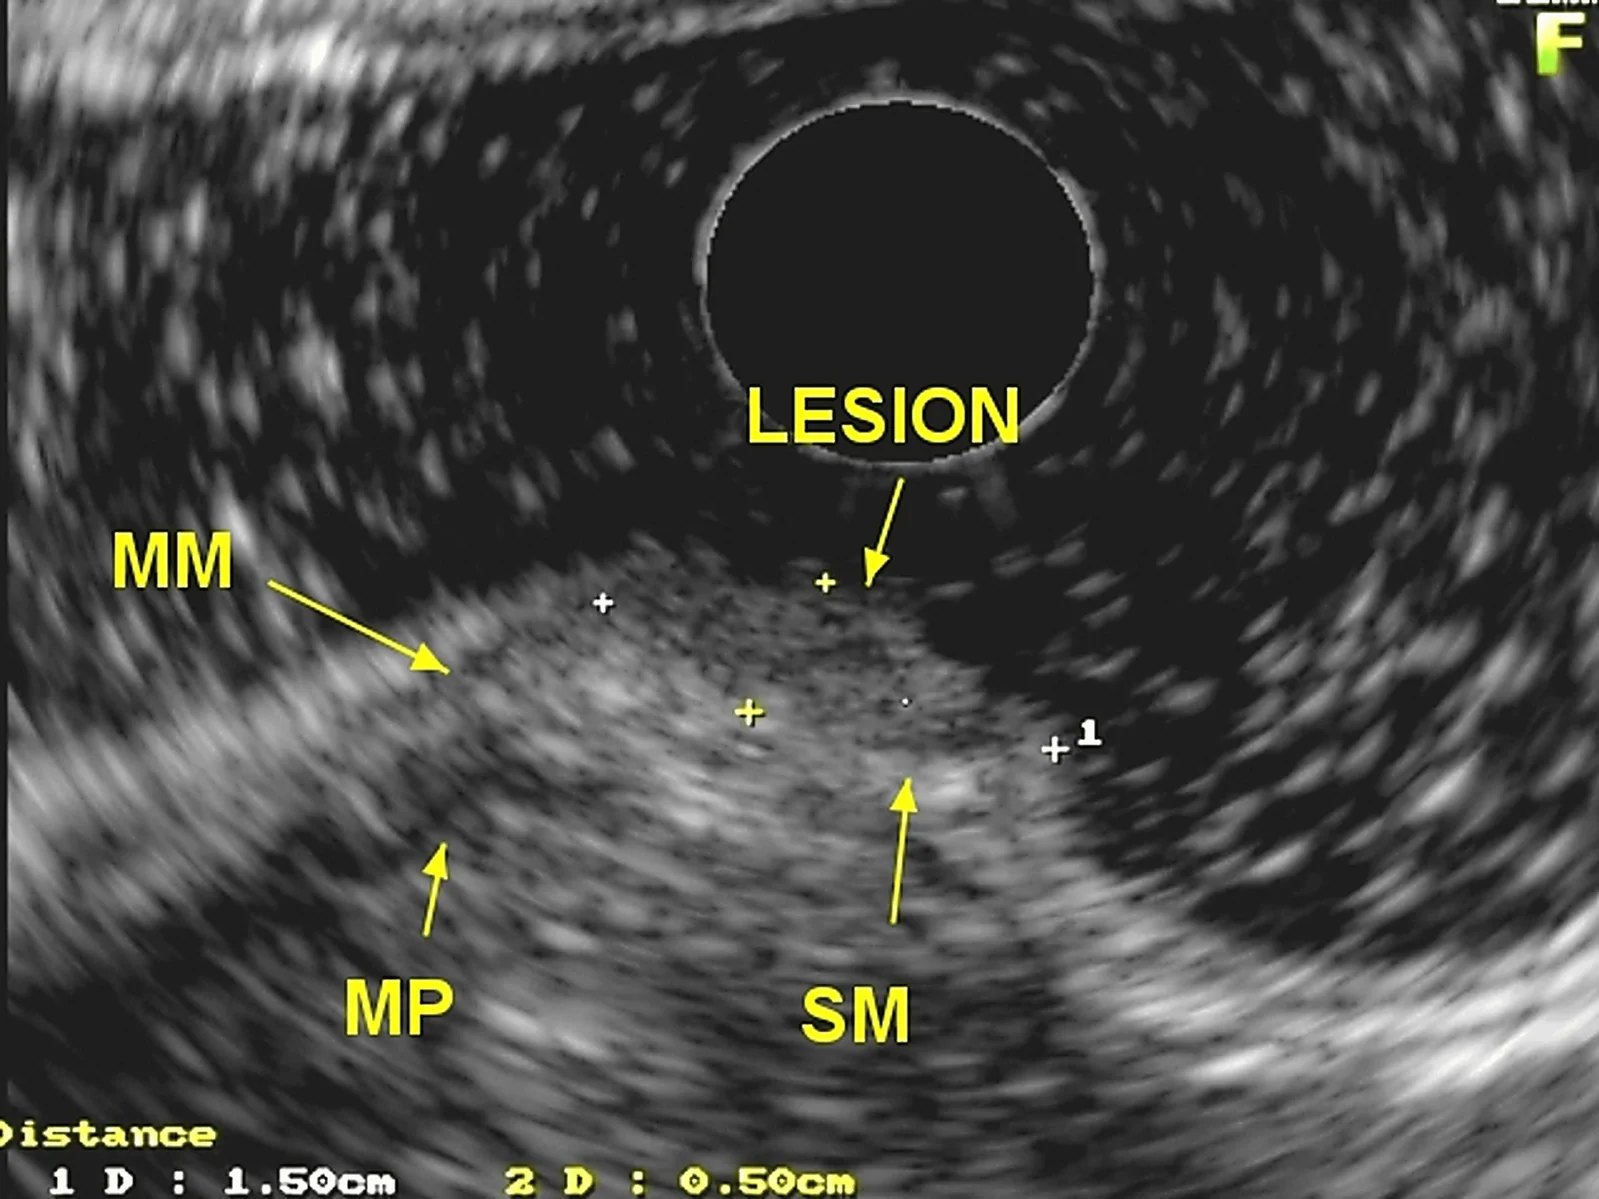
Radial EUS showing a hypoechoic gastric lesion involving the mucosa and MM, with focal contact with the SM; the MP is intact Electronic caliper measurements: 1.50 × 0.50 cm MM: muscularis mucosae, SM: superficial submucosa, MP: muscularis propria, EUS: endoscopic ultrasonography
